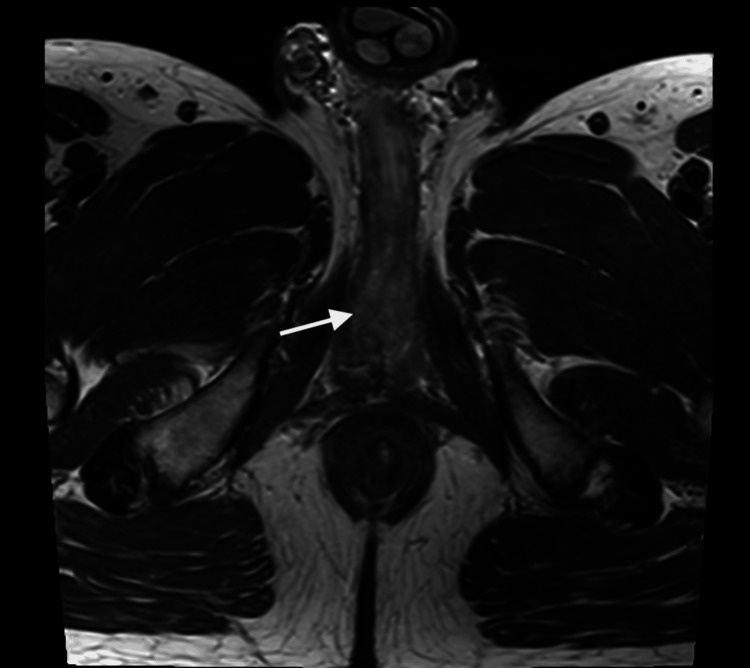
Urethral mass on MRI axial view (white arrow).
